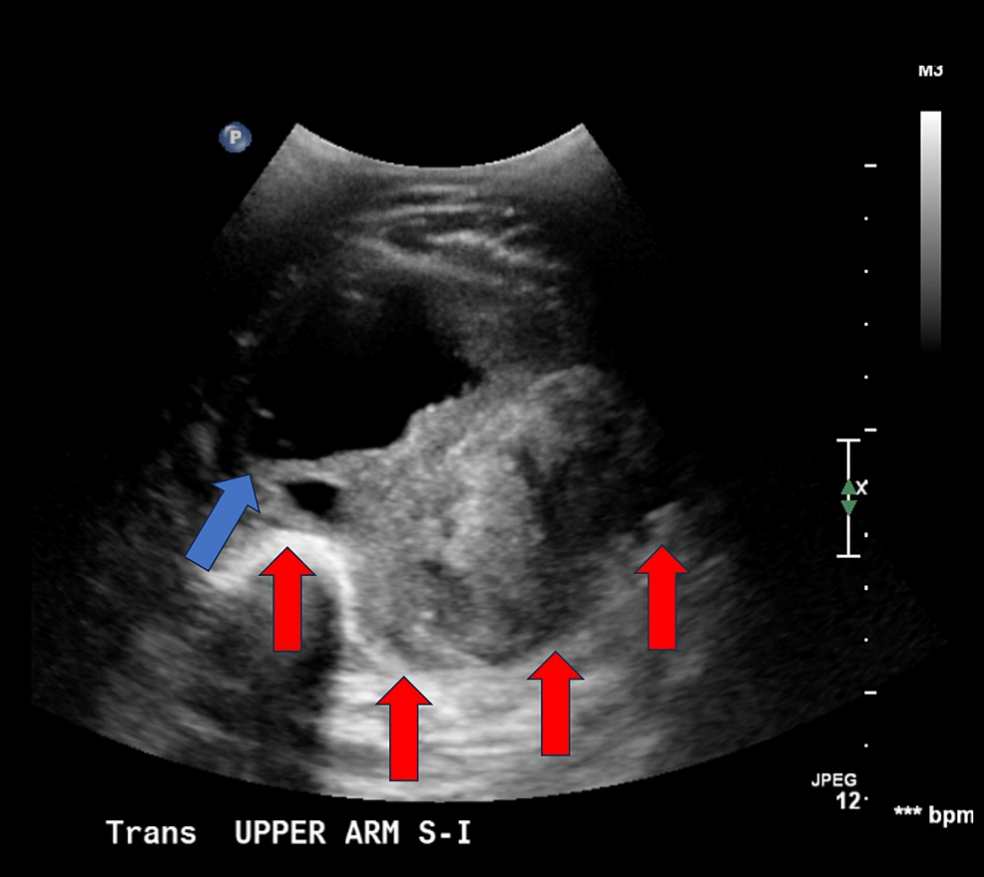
Ultrasound displaying a heterogenous nonvascular mass measuring 13.4 cm× 8.6 cm × 8.1 cm (red arrows indicating borders of the pseudocapsule, blue arrows indicating peritumoral edematous fluid accumulation). The radiologist reported, “negative for deep venous thrombosis with the primary differential consideration of evolving hematoma and myositis.”
